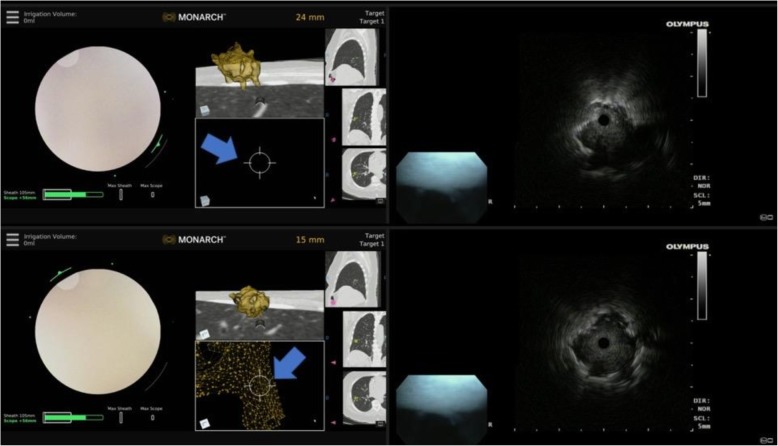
Eccentric and concentric REBUS image patterns during robotic bronchoscopy. Top panel: at 24 mm away from the nodule, the REBUS probe was advanced and only an eccentric pattern was obtained; note that the target is not seen on the Monarch EMN display monitor (blue arrow). Bottom panel: the scope is advanced to 15 mm away from the lesion, and the REBUS monitor now shows a concentric pattern (associated with higher diagnostic yield). Note that the nodule is now also seen on the Monarch EMN display monitor (blue arrow). REBUS: radial probe ultrasound; EMN: electromagnetic navigation
